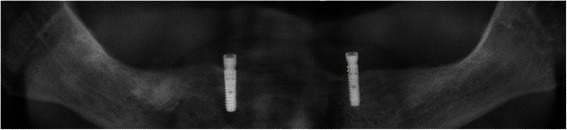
Radiograph showing test and control implant in the interforaminal region. The analysis was performed by an independent investigator using ImageJ software
